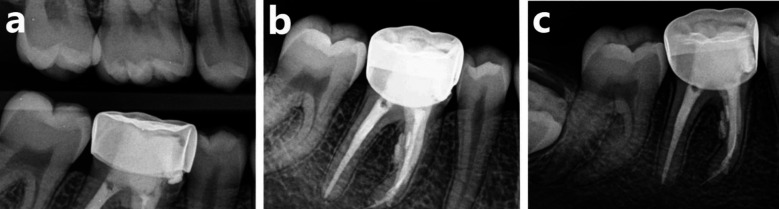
Follow up Radiographs at 18 months (a) Bitewing radiograph, (b) IOPA showing complete furcal healing, (c) IOPA showing complete periapical healing.
